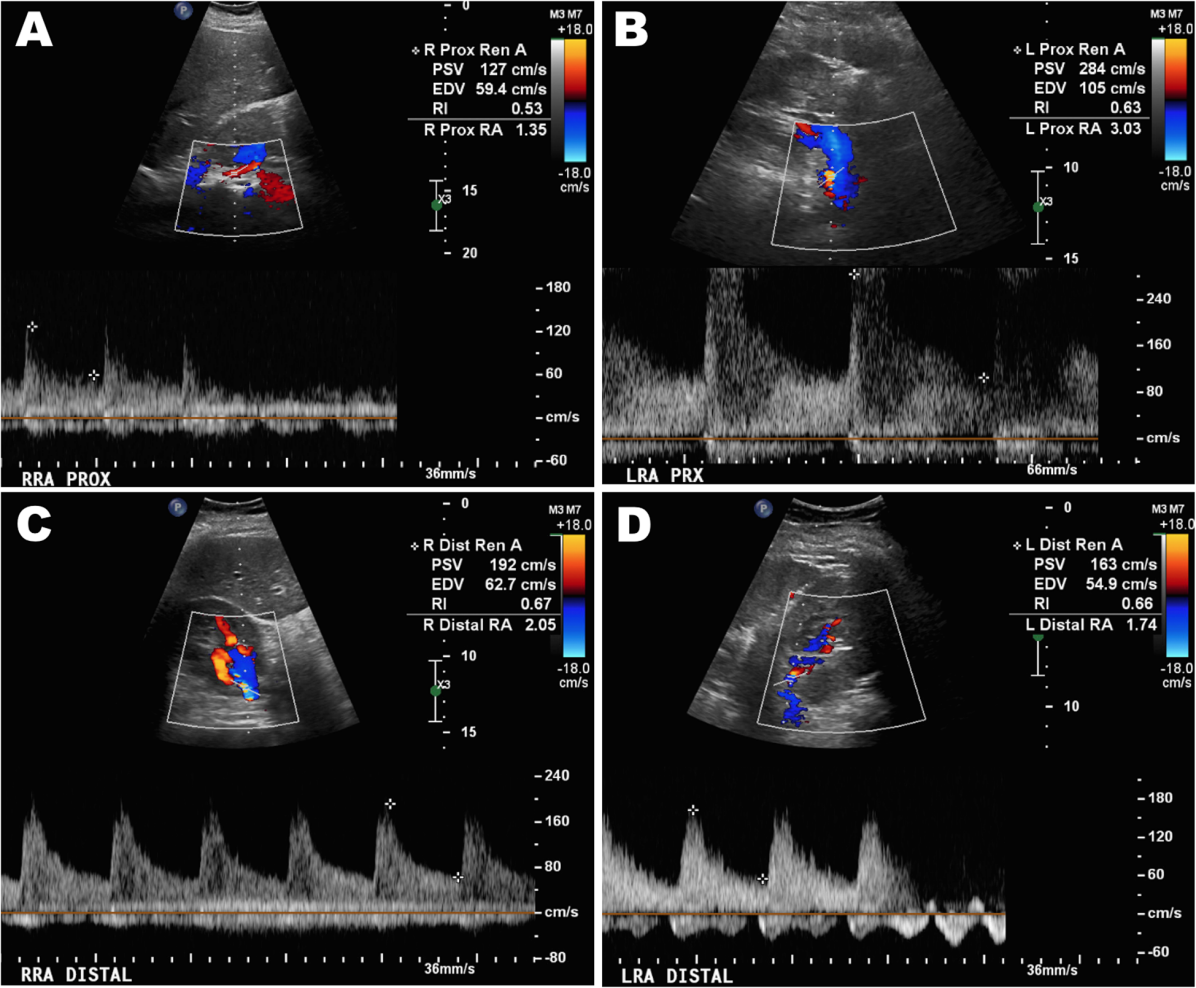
Doppler ultrasonography with peak systolic velocities (PSV) of the right proximal (a), left proximal (b), right distal (c) and left distal (d) renal arteries [Normal PSV < 180 cm/s]
